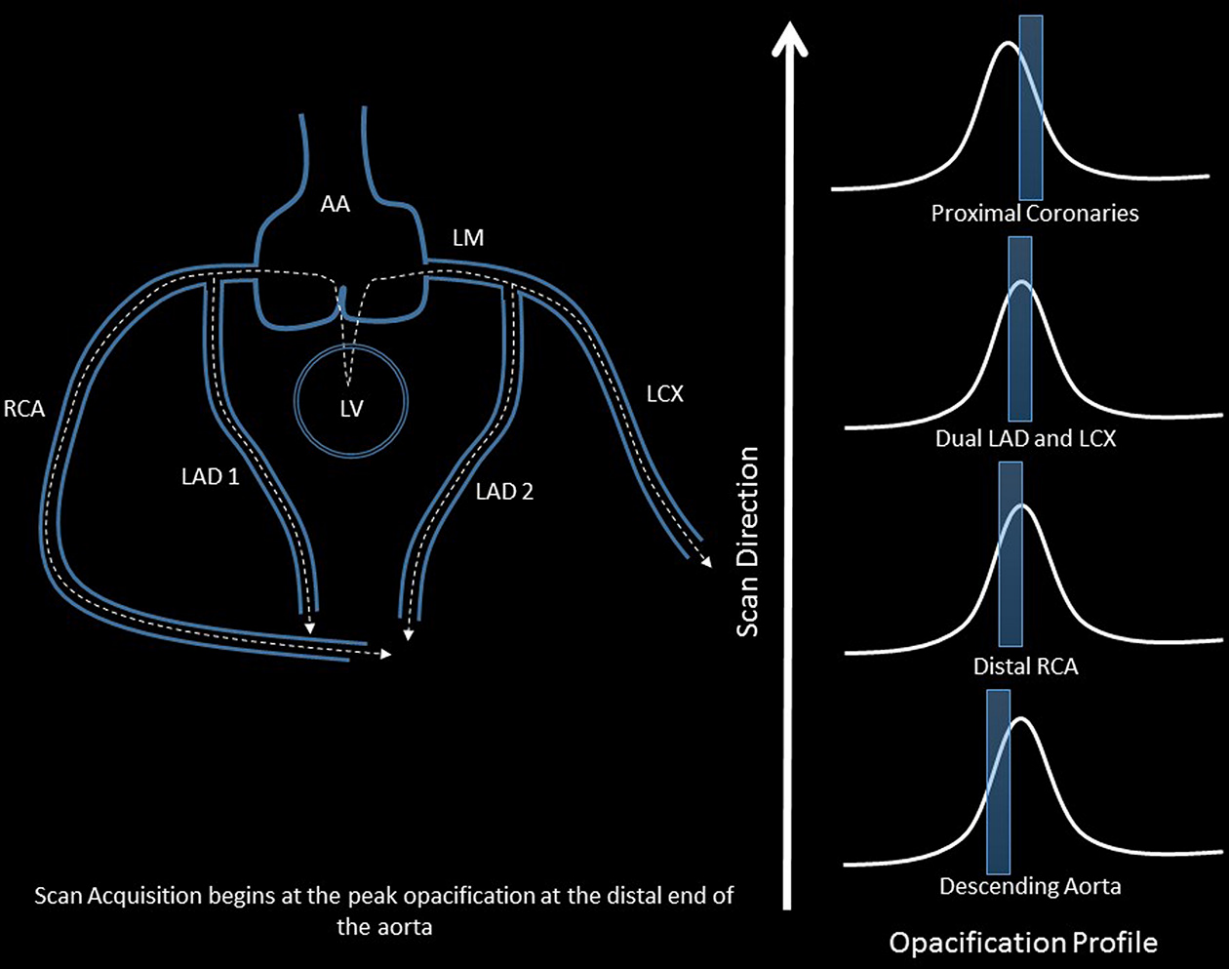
This image demonstrates the optimal time to begin the CTA acquisition relative to the contrast media opacification profile. The dotted lines with the arrows demonstrate the arterial blood flow direction, whereas the scan acquisition is in the caudocranial direction (long white arrow). The opacification profiles demonstrate the scan phase relative to the contrast opacification curve at each anatomical location. The first curve demonstrates the scan acquisition box at the level of descending aorta, whereas the last curve (relative to the end of the scan direction), shows the proximal coronary artery scan phase during the opacification profile. AA, ascending aorta; CTA, CT angiography; LAD, left anterior descending artery; LCX, left circumflex artery; LM, left main coronary artery.
